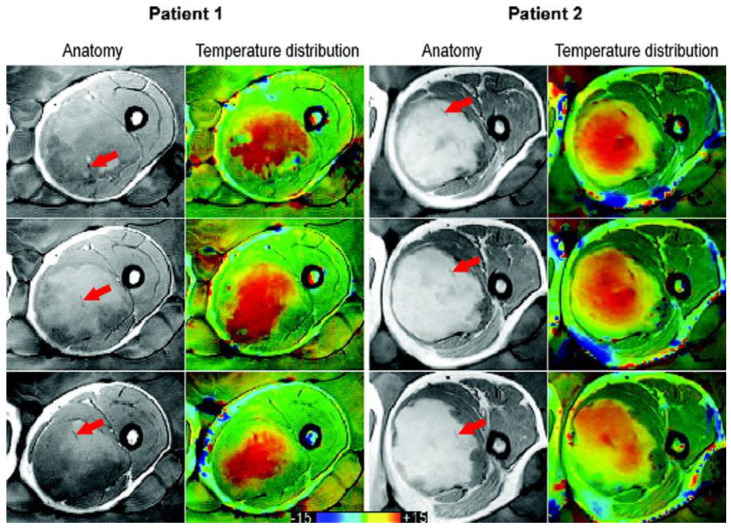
Taken from Gellermann et al. [21] and re-printed with permission from John Wiley and Sons. Example of anatomy with thermal mapping catheters (red arrows) for two patients with corresponding MR temperature distributions for three different slices. The images were acquired with a T1-weighted gradient-echo sequence. (The arrows were superimposed on the original image for clarity.)
